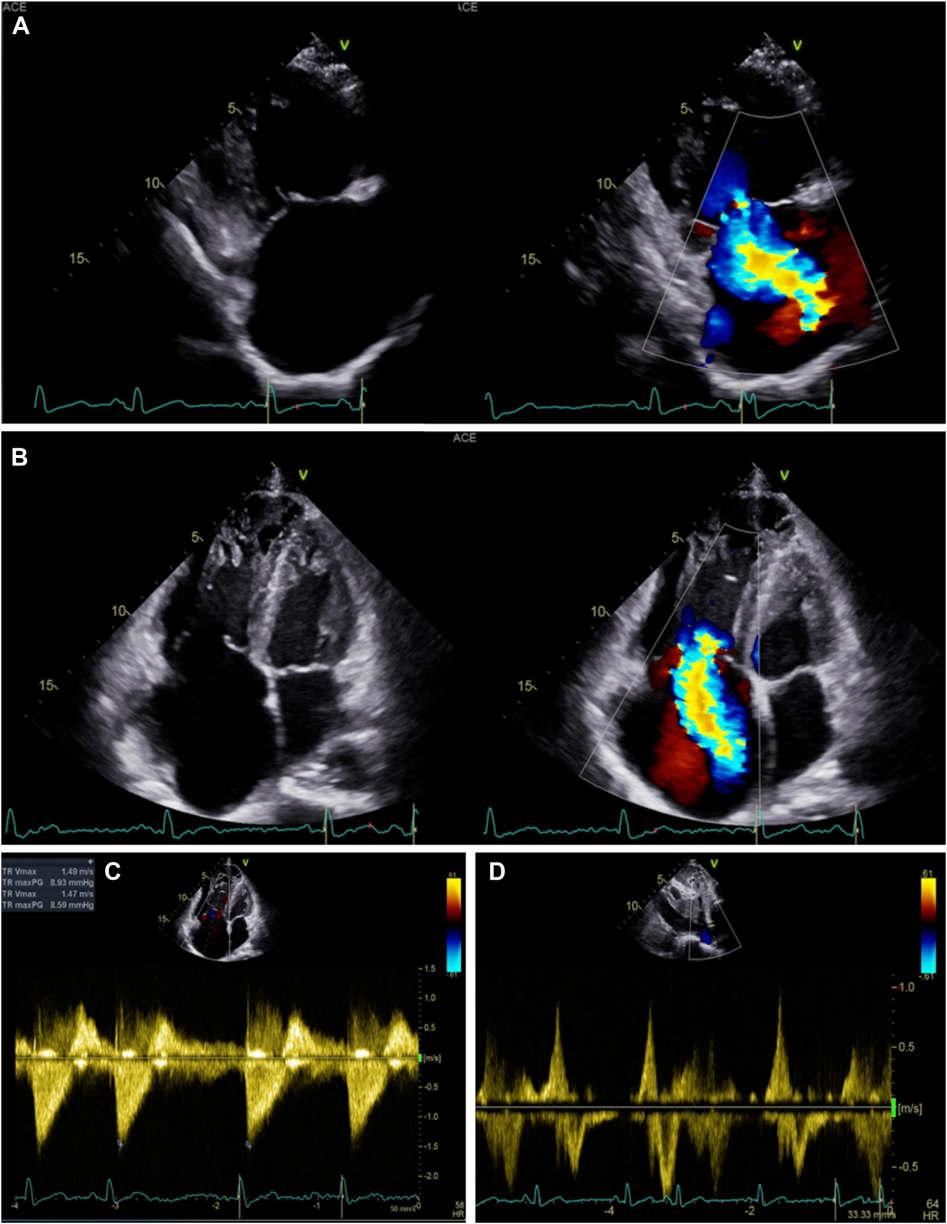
Two-dimensional TTE, RV inflow (A) and apical 4-chamber (B) views, without (*left*) and with (*right*) color-flow Doppler, demonstrates the dilated right heart and severe TR. Continuous-wave Doppler through the TV (C) demonstrates a triangular-shaped, low-velocity spectral Doppler pattern of the severe TR. Pulsed-wave Doppler within the superior hepatic vein (D) demonstrates systolic flow reversal.
